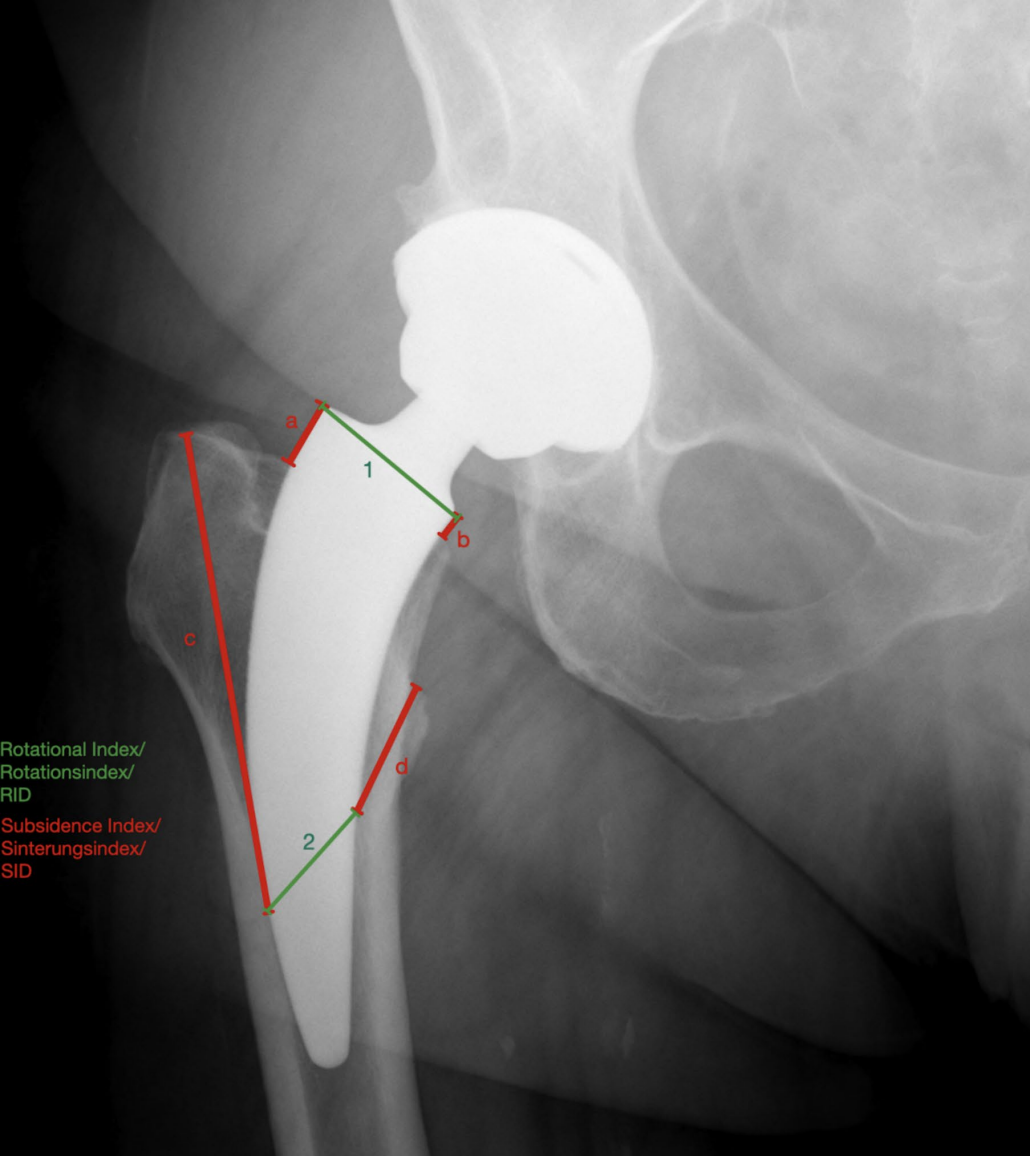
Example of Subsidence- and Rotational-Index shown on conventional standing pelvic radiograph; a upper corner of the short stem just below the artificial femoral neck and ending at the point of entry of the short stem into the femur; b same distance as (a) but on the opposite side of the short stem; c distinct mark on the lateral side of the short stem, which is defined by a change in coating, to a specific point above the trochanter major; d distinct mark which is defined by a change in coating but on the medial side of the short stem to a specific point at the trochanter minor; (1) diameter just below the artificial femoral neck; (2) medial mark to the lateral mark on the short stem
